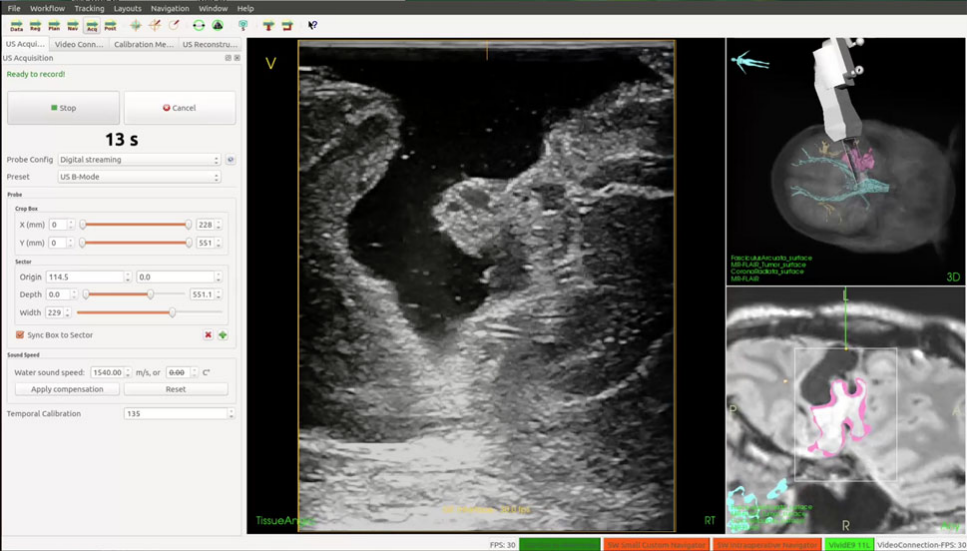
Ultrasound acquisition during brain tumor surgery
